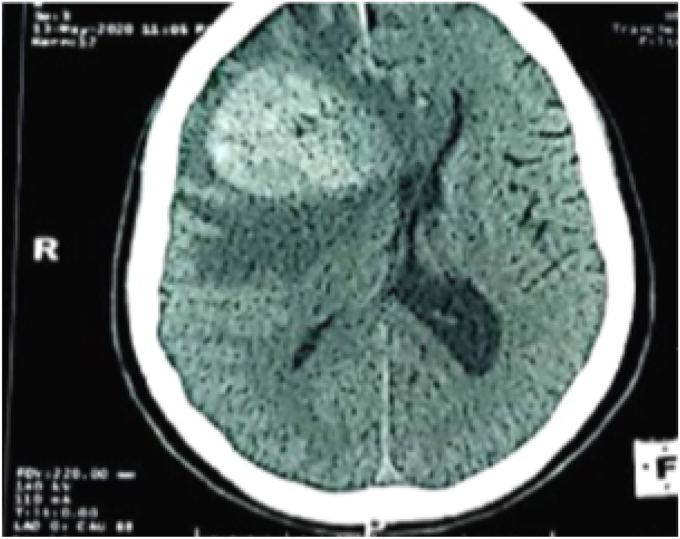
CT SCAN: right fronto-parietal formation that was spontaneously hyperdense and surrounded by a peri-lesional edema.
